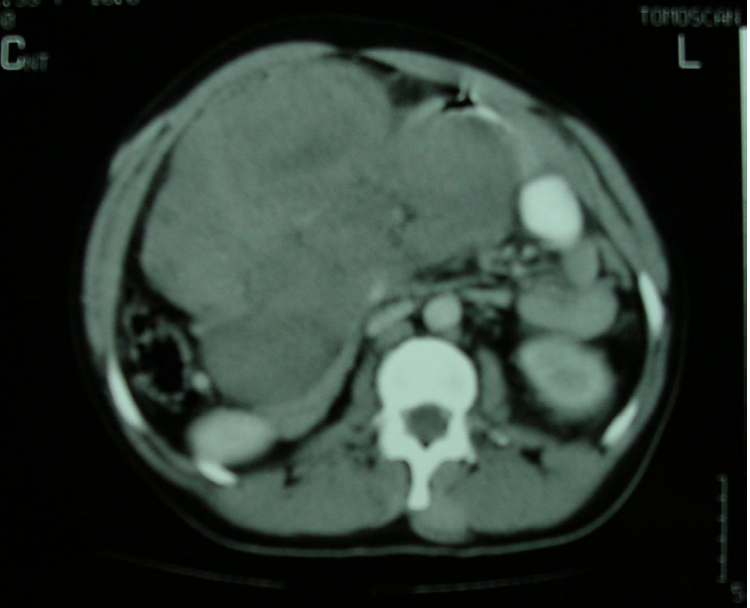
CT scan of the abdomen with contrast media reveals a large-size intra-abdominal mass displacing the adjacent structures. In the same scan a suspicious lesion is identified in the right adrenal. In some rare cases desmoid tumors may co-exist with adrenal or thyroid carcinomas and adrenal adenomas.
